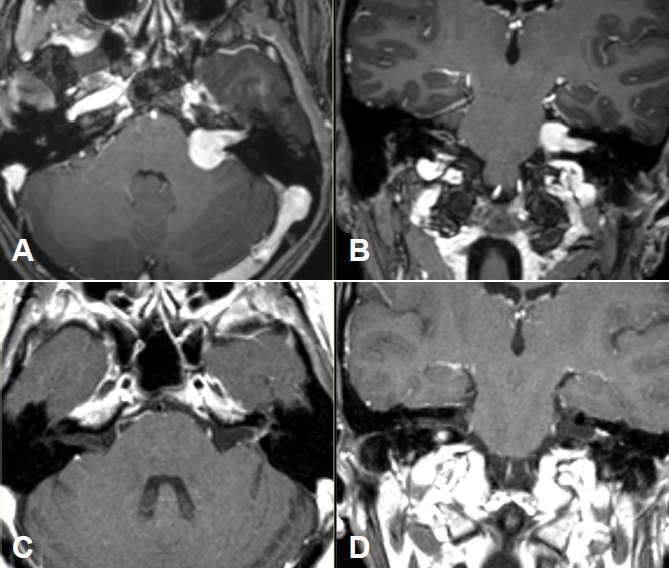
Preoperative MRI, T1-w + CM; A transversal; B. Postoperative MRI, T1-w + CM; C transversal, D coronal.
